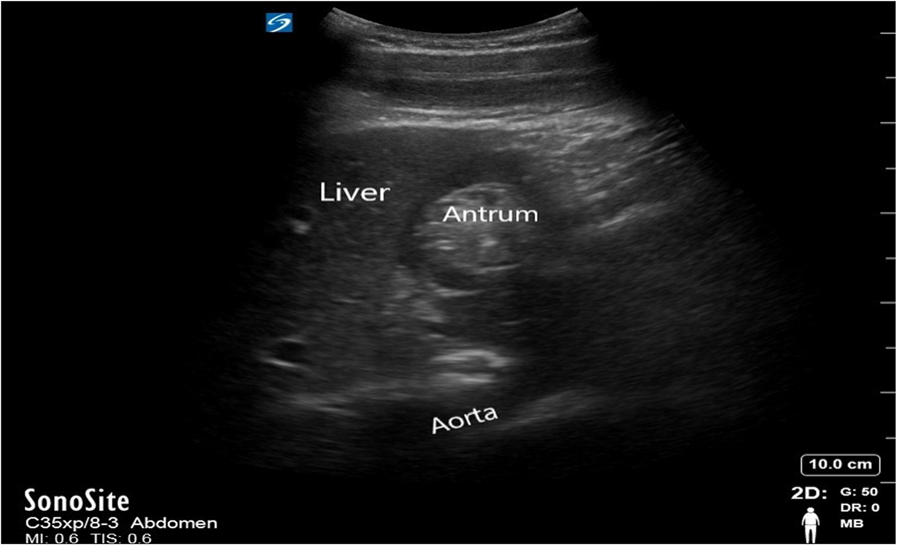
Example of a gastric ultrasonographic image. The antrum was located superficially between the left lobe of the liver anteriorly and the pancreas posteriorly in a sagittal or parasagittal scanning plane in the epigastrium. Important vascular landmarks including the inferior vena cava (IVC) and the superior mesenteric vein was marked the standard scanning plane of the antrum. Antralcross-sectional area (CSA) can be measured by using two perpendicular diameters (antero-posterior diameter and craniocaudal diameter) and the formula of the area of an ellipse
